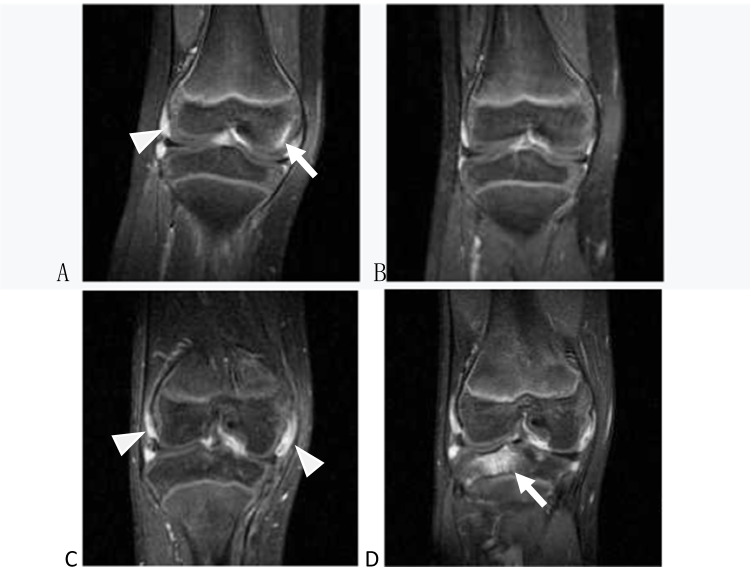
A, B 7-year-old girl, coronal T1-W FS Gd-enhanced MRI. A BME in the medial femoral condyle (arrow). Note the accompanying synovitis (arrowhead). B BME disappeared at the follow-up visit 6 months after standard treatment. C, D 11-year-old boy, coronal T1-W FS Gd-enhanced MRI. C No BME was visible at the initial MRI examination, but the synovitis was clear (arrowhead). D After 10 months with reduced dosage even withdrawal, BME appeared in the lateral tibial plateau (arrow)
